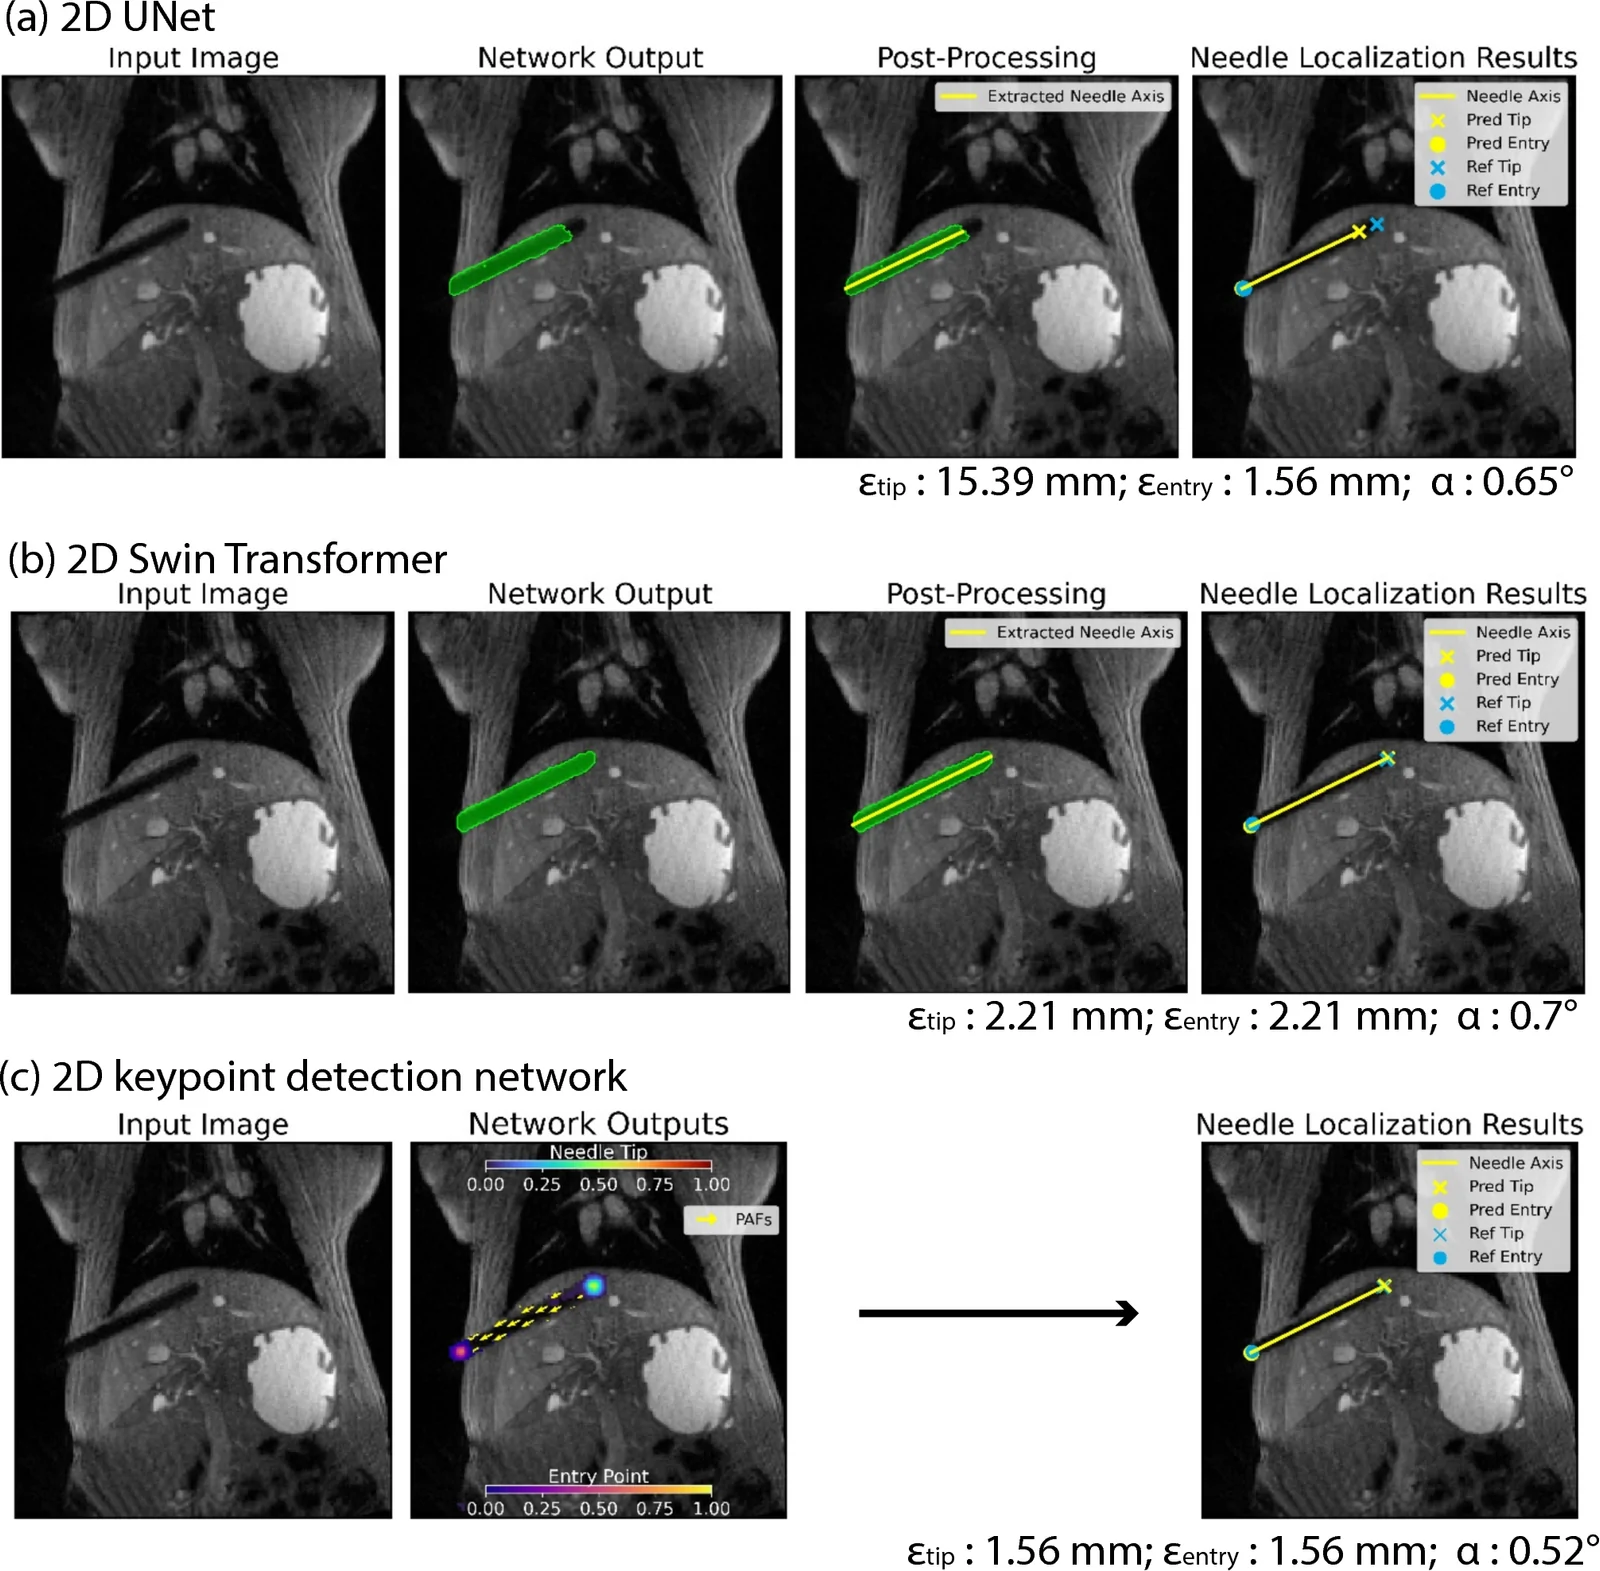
Example of needle localization on a single-slice controlled-breathing (SS-CB) MR image using a 2D UNet, b 2D Swin Transformer, and c 2D keypoint detection network. Reference needle tip and entry point annotations were shown as a blue cross and dot. The prediction results were shown in yellow. The 2D keypoint network directly predicted the locations of the needle tip and entry point without the need for an additional post-processing step of needle axis and coordinate extraction
